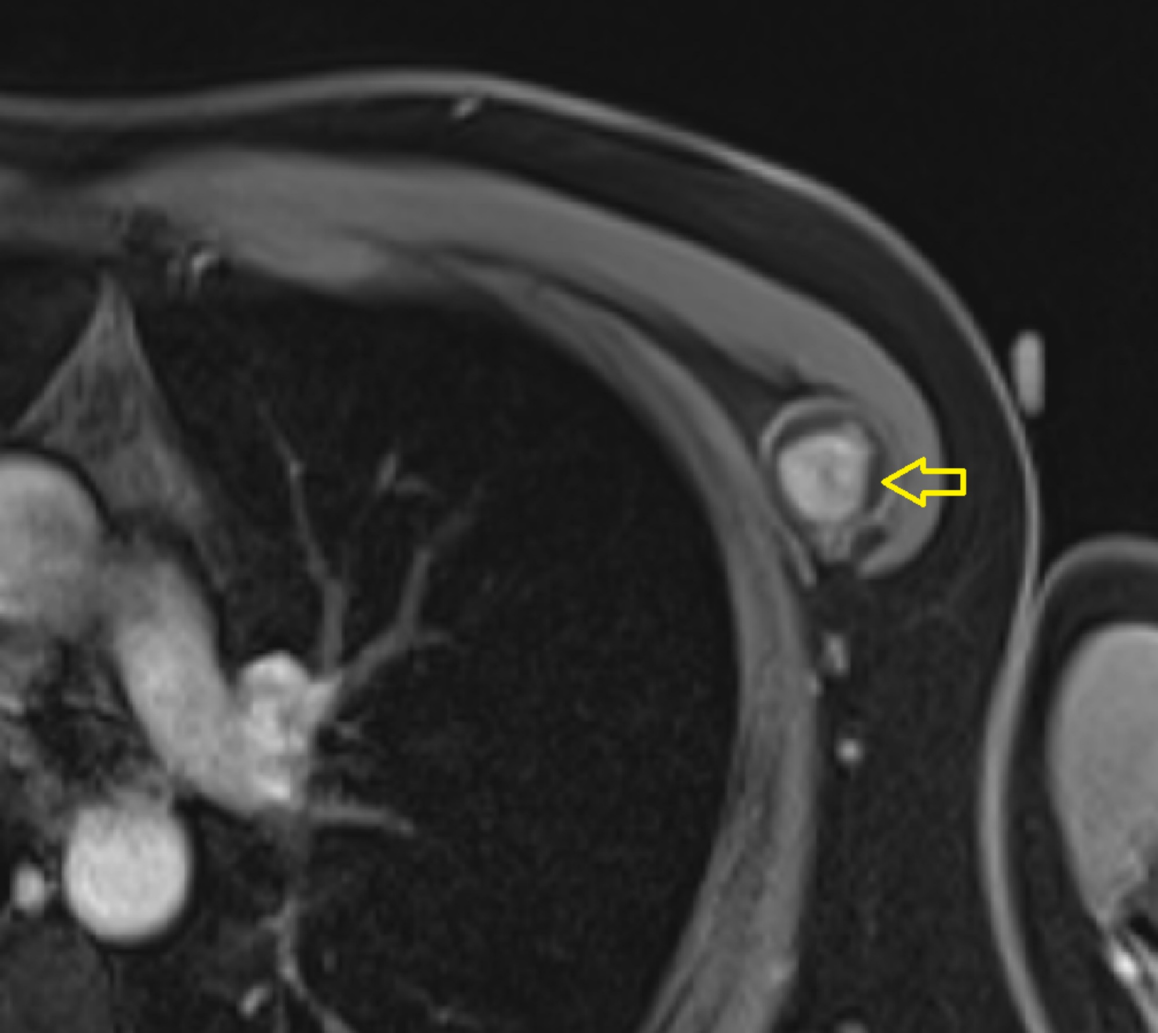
T1-weighted post-contrast MRI Well-defined lesion (arrow) with post-contrast enhancement. MRI: magnetic resonance imaging
